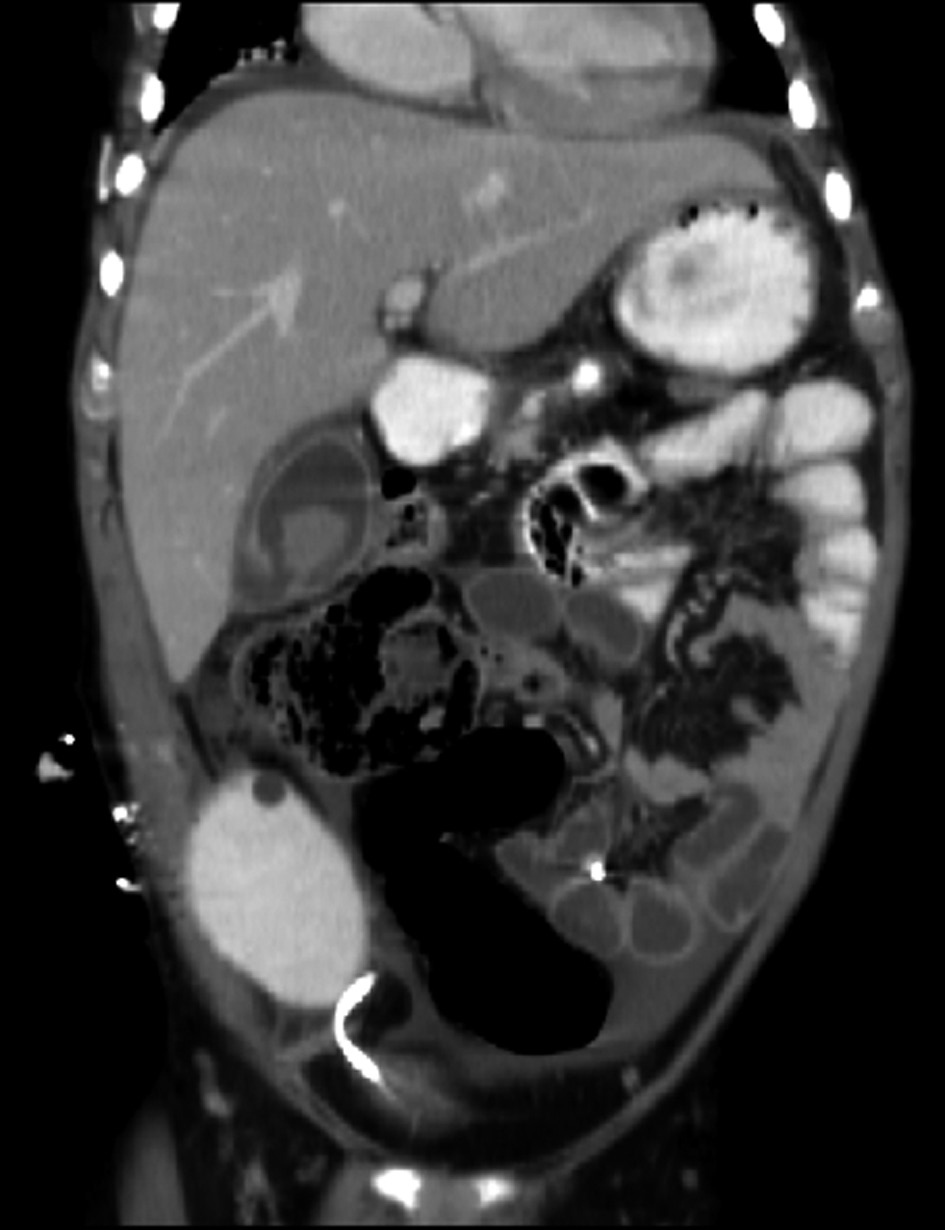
CT scan showing perforated GB: the “HOLE” sign
